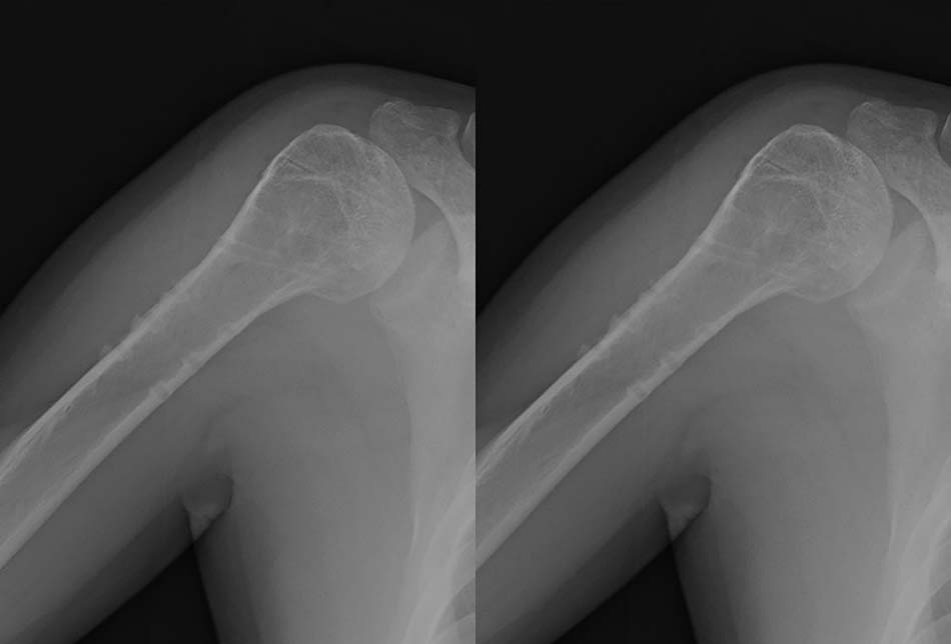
Post-implant removal X-ray showing that the alignment had been maintained.
